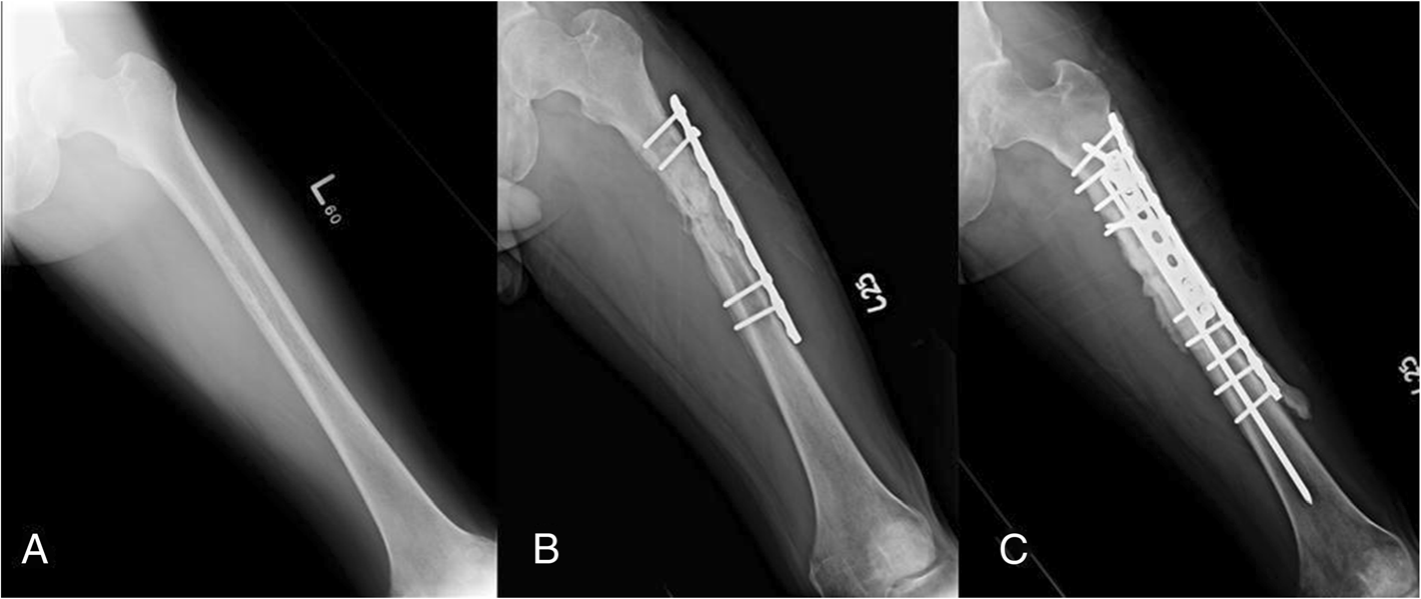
A 33-year-old man who complained of nocturnal thigh pain and weight loss noted over six months. (A) A plain film of the femur showing moth-eaten osteolytic lesion with cortical destruction and laminated periosteal reaction. (B) A plain film taken six weeks after prophylactic fixation and debridement surgery showing recurrent osteomyelitis and progressive destruction of the medial cortex with pathological fracture. (C) A plain film taken one year after the final surgery showing bony healing without recurrence.
